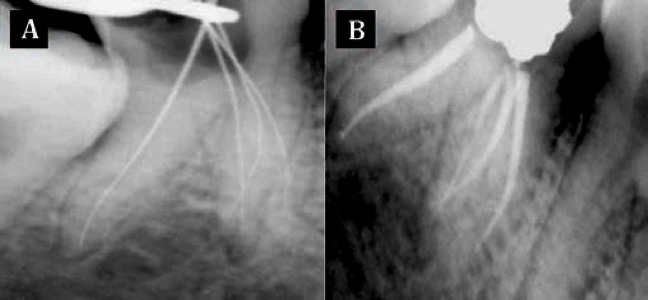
A) Working length confirmatory radiograph; and B) immediate post-obturation radiograph
